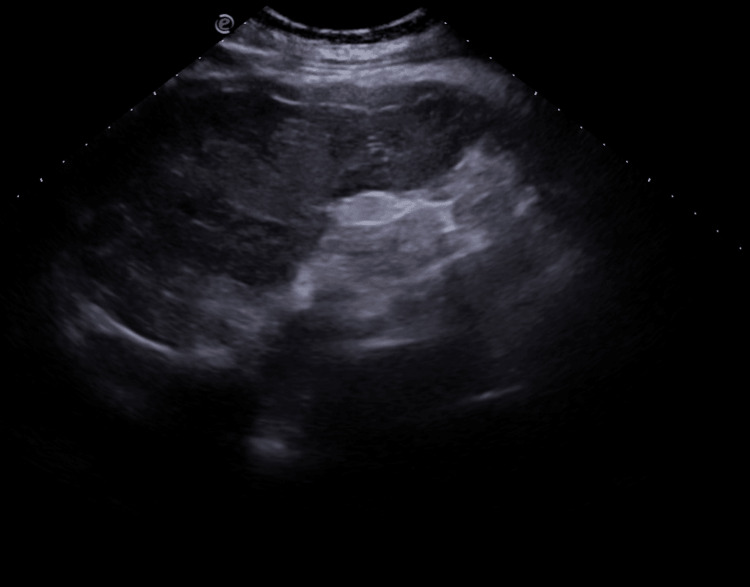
Ultrasonography of the abdomen Splenomegaly, with ill-defined confluent hypoechoic areas, is seen predominantly involving the peripheral portion of the spleen. The splenic capsule appears irregular, thickened, and echogenic with areas of subtle posterior acoustic shadowing suggestive of soft calcification. Associated hypoechoic areas are seen in the subcapsular region.
